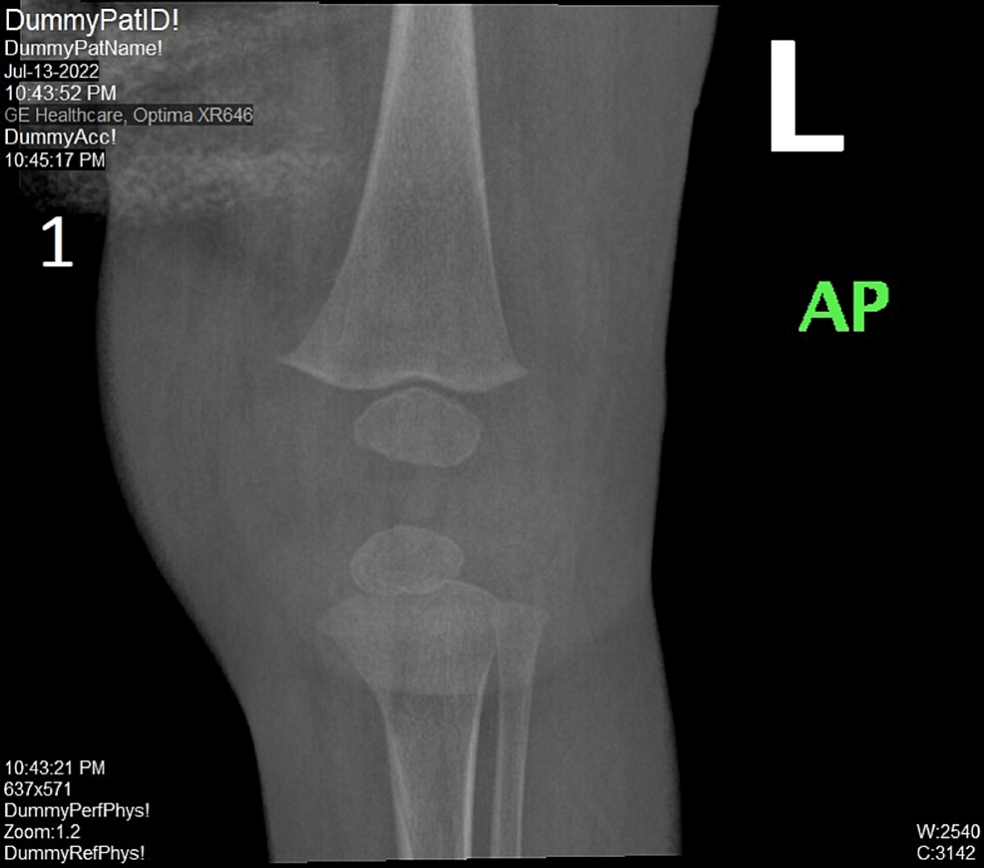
X-ray of the left knee joint AP view This figure shows the expansion of the joint capsule, normal alignment, and no fracture lines. AP: Anterior-posterior.
